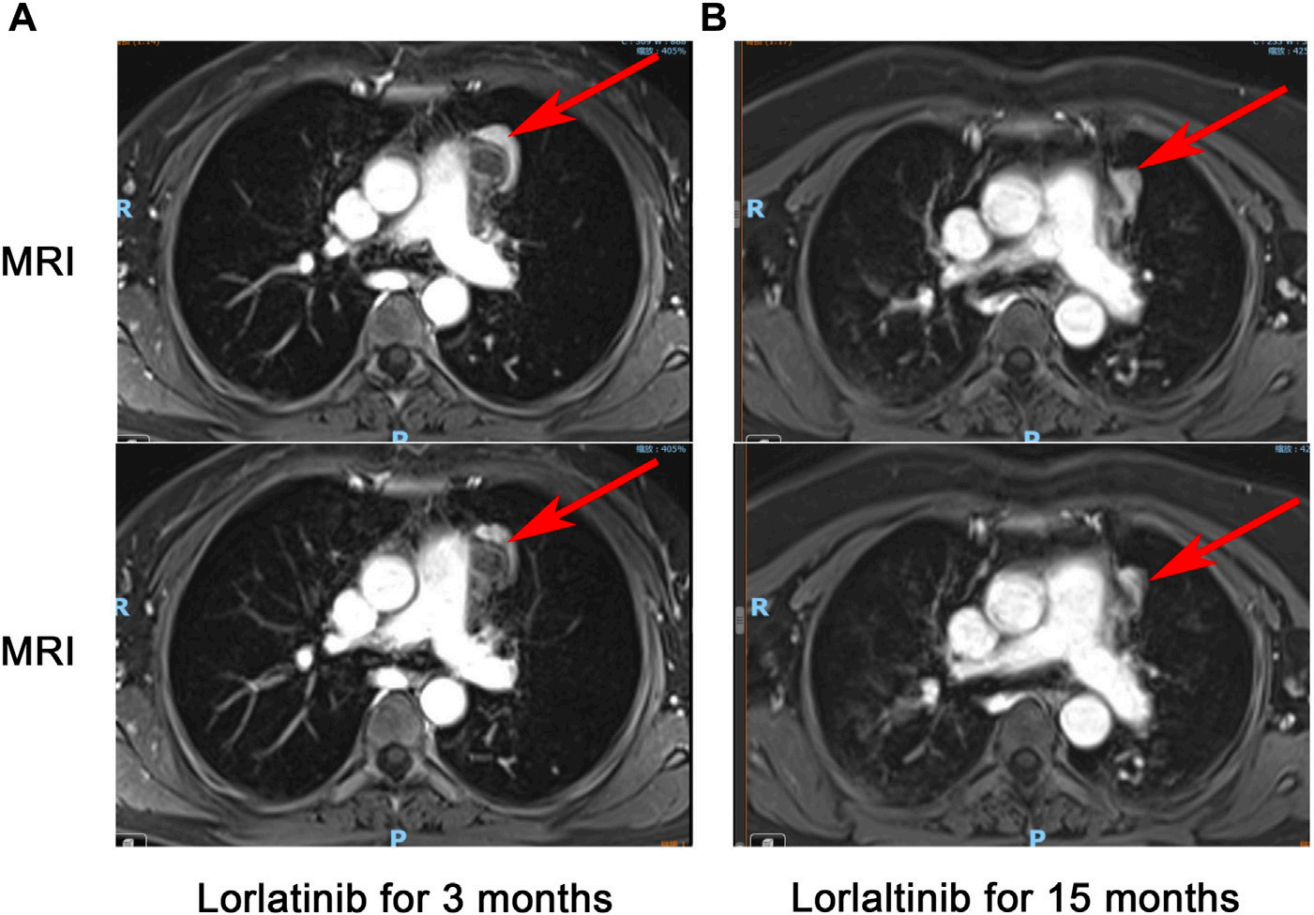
Chest-enhanced MRI with contrast: The tumor infiltrates the mediastinal vessels after 3 months of treatment with Lorlatinib (A), tumor separates from the surrounding blood vessels after 15 months of treatment with Lorlatinib (B).
